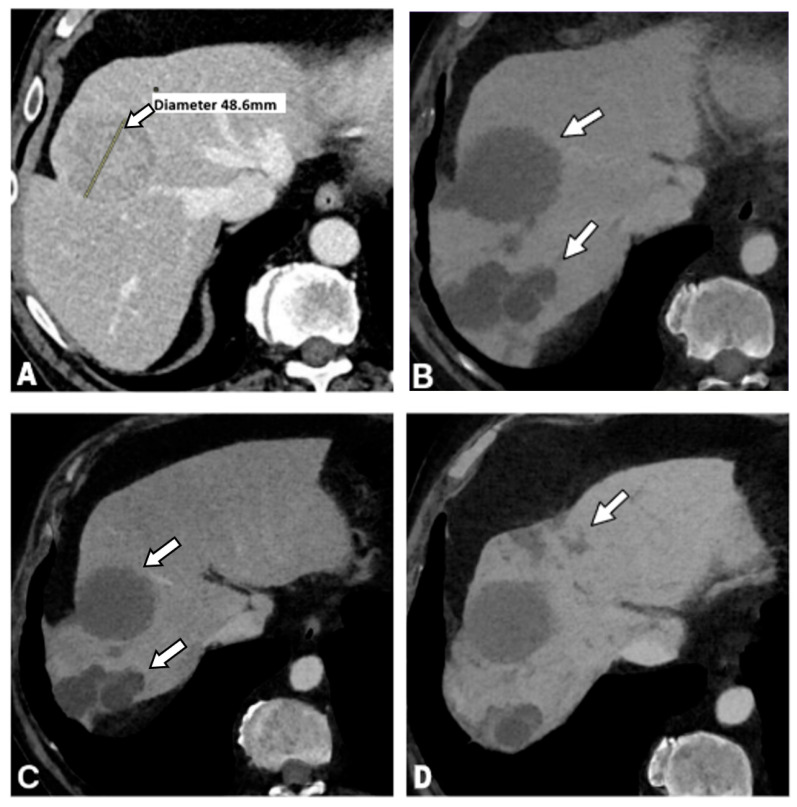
Evolution of ischemic cholangiopathy after TACE in patient No. 12 from Table 1 with HCC in a cirrhotic liver. (A) Baseline contrast-enhanced CT shows a 48 mm HCC (arrow) before treatment. (B) MinIP CT reconstruction obtained 3 months after TACE demonstrates two large bilomas (arrows) developing in the treated hepatic parenchyma. (C) At 12 months, CT shows slight regression of both bilomas (arrows). (D) On subsequent follow-up after 24 months and an additional TACE procedure, the bilomas are unchanged. while new focal bile duct dilatation (arrow) is visible.
